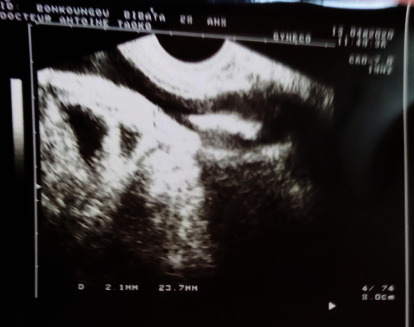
Hyperechoic image of intrauterine device in the bladder on pelvic ultrasonography.
